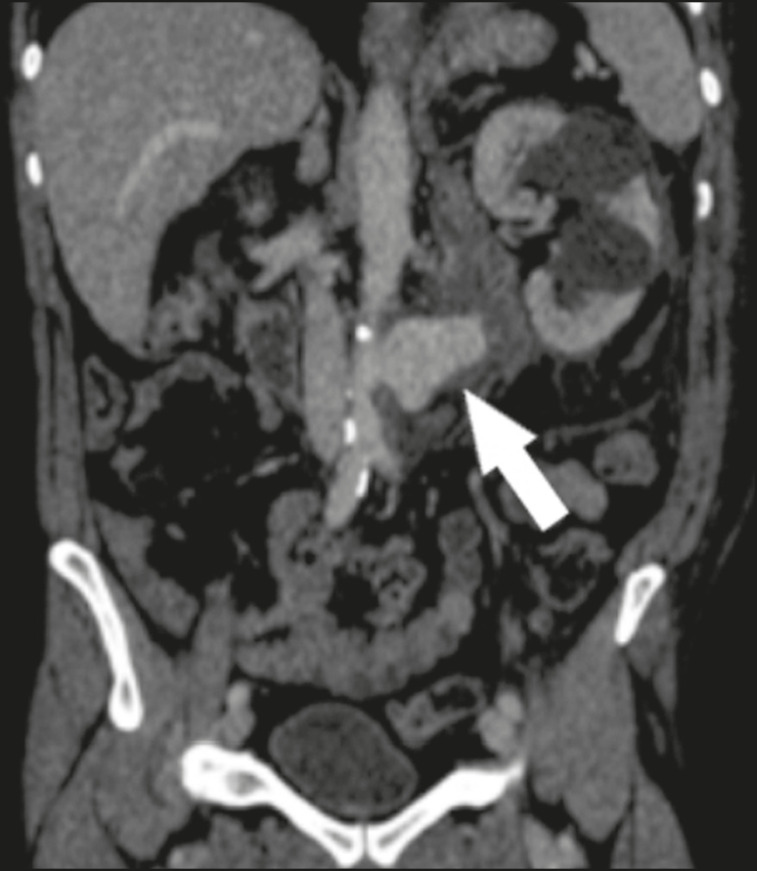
Coronal CT angiography of the abdomen with iodinated contrast, in the arterial phase, showing pseudoaneurysm to the left of the infrarenal abdominal aorta (arrow).
